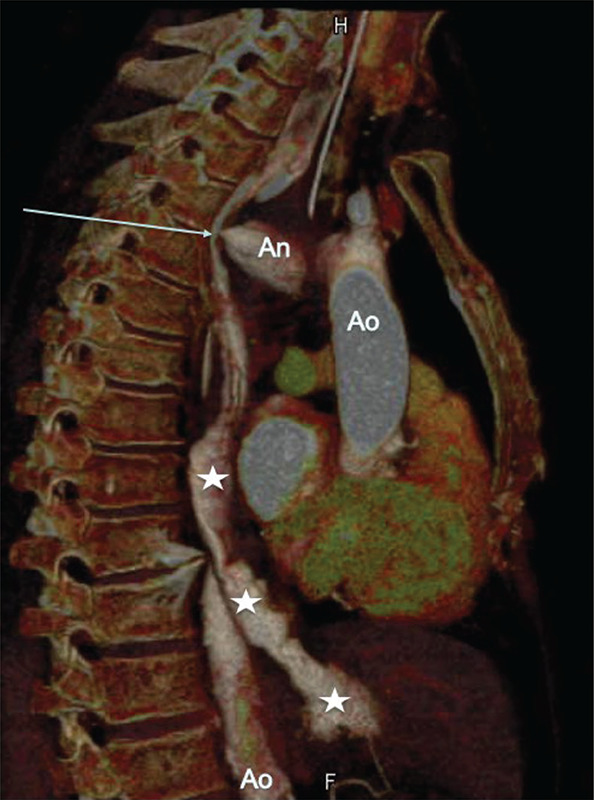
Three‑dimensional (3D) volume‑rendering technique (VRT) image of the CTA in sagittal orientation showing the fistula (arrow) between the aneurysm (An) and contrast material within the esophagus. Aorta (Ao). Esophagus (white stars).
